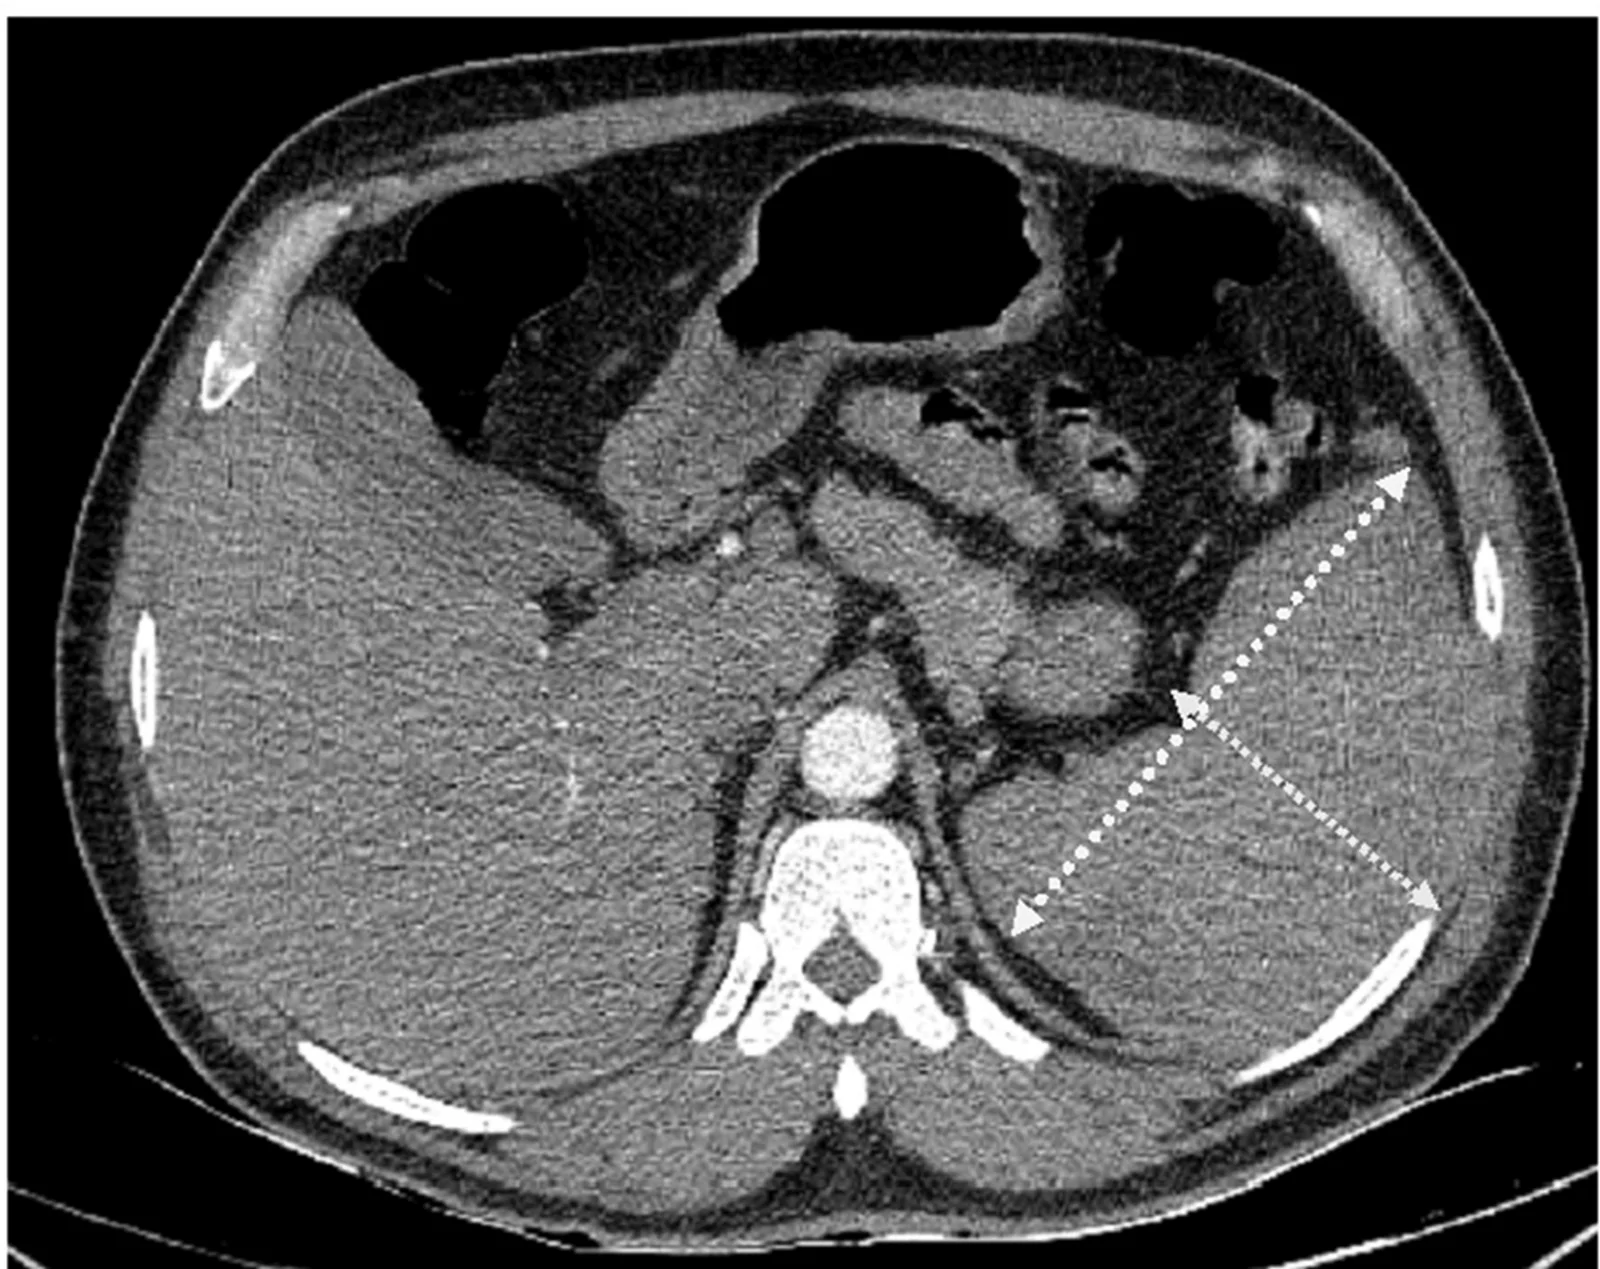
Contrast-enhanced axial computed tomography image of the abdomen demonstrating splenomegaly The dotted measurement lines demonstrate splenic dimensions of approximately 15.1 cm along the long axis and 6.8 cm along the short axis.
